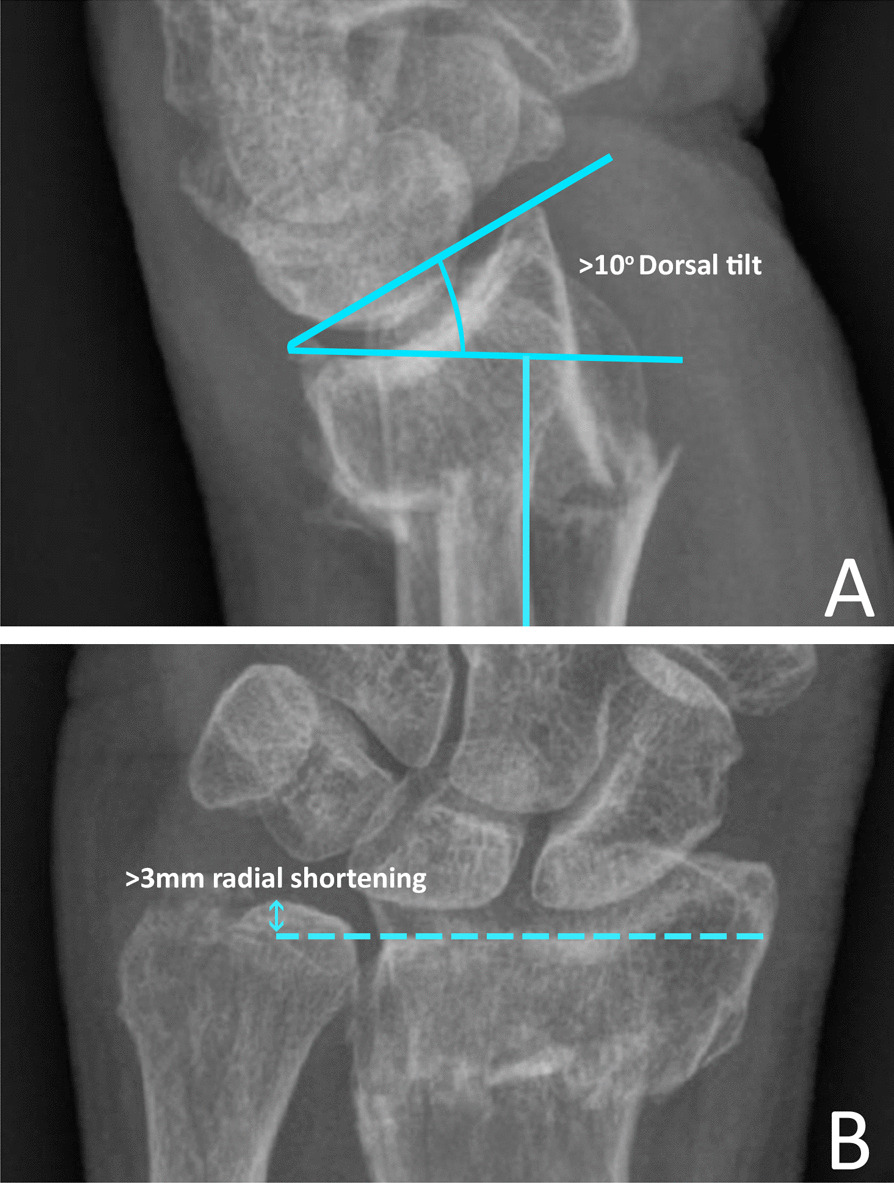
Fracture displacement measured according to the AAOS guideline. A The degree of angulation (volar or dorsal) was measured on the lateral view radiographs. This value represents the angle between a line along the distal radial articular surface and the line perpendicular to the longitudinal axis of the radius [10]. B Radial shortening was measured on the posteroanterior radiograph and refers to the distance between the carpal joint surface of the radius and the most distal part of the ulnar articular surface [14]
